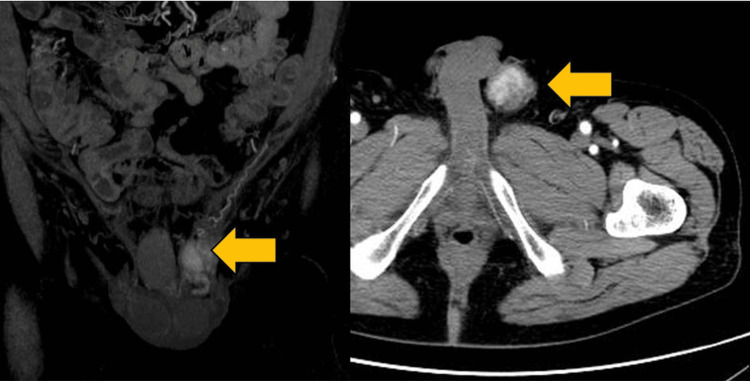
Coronal and axial enhanced CT images showing a solid tumor in the left spermatic cord (yellow arrows). Coronal (left) and axial (right) enhanced CT scans reveal a well-defined, solid mass (yellow arrows) in the left spermatic cord. The tumor appears hyperdense relative to the surrounding soft tissue structures.
